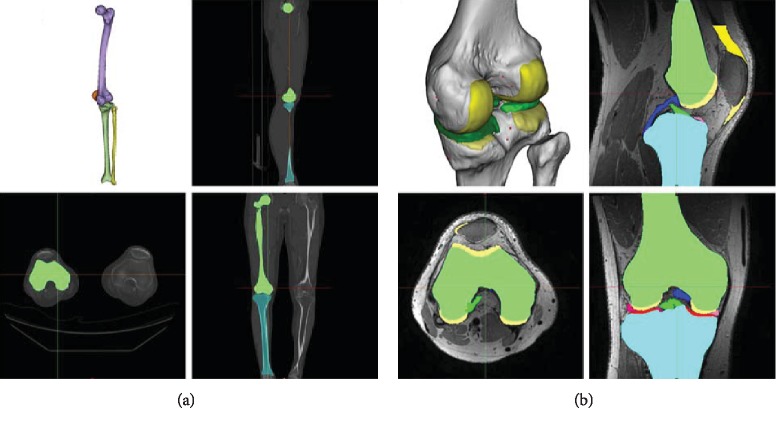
3D medical imaging data of (a) CT and (b) MRI used in the development of the PS UKA models.
